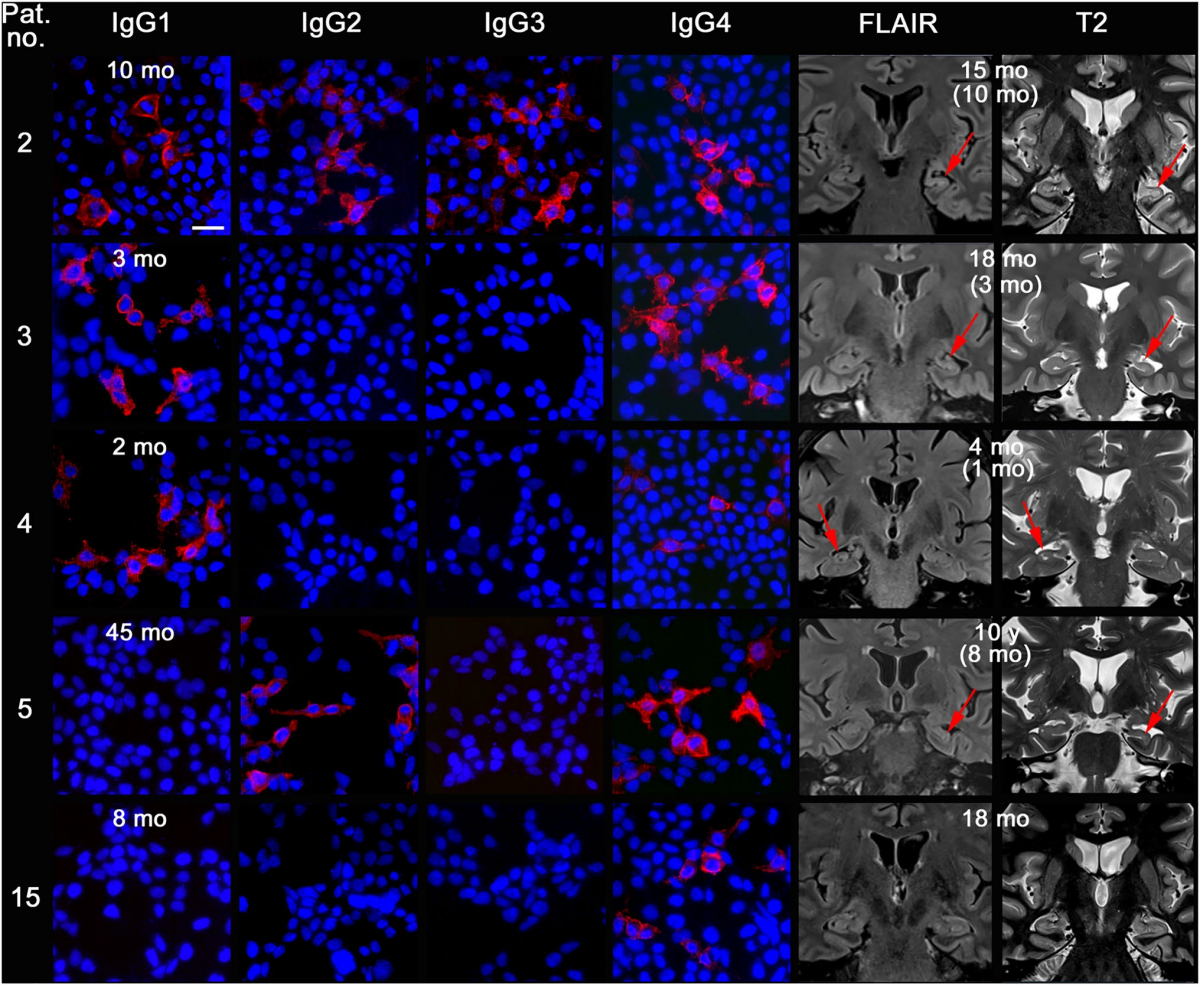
Five exemplary anti-leucine-rich, glioma inactivated 1-(LGI1-)immunoglobulin G subclass studies on HEK cells transfected with LGI1 and the patients’ most recent coronal MRI. The patient numbers in the first column are those in Table 2. MRI sections go through the hippocampi. Affected hippocampi are marked by arrows. Fluid-attenuated inversion recovery (FLAIR) images emphasize the increased signal in the affected hippocampi, while T2 images highlight the hippocampal atrophy. The time specifications give the latencies from disease onset to the respective depicted diagnostics. In the MRI columns, time specifications in brackets indicate when hippocampal sclerosis was detectable for the first time. Bar: 15 µm, valid for all cell-based assays
